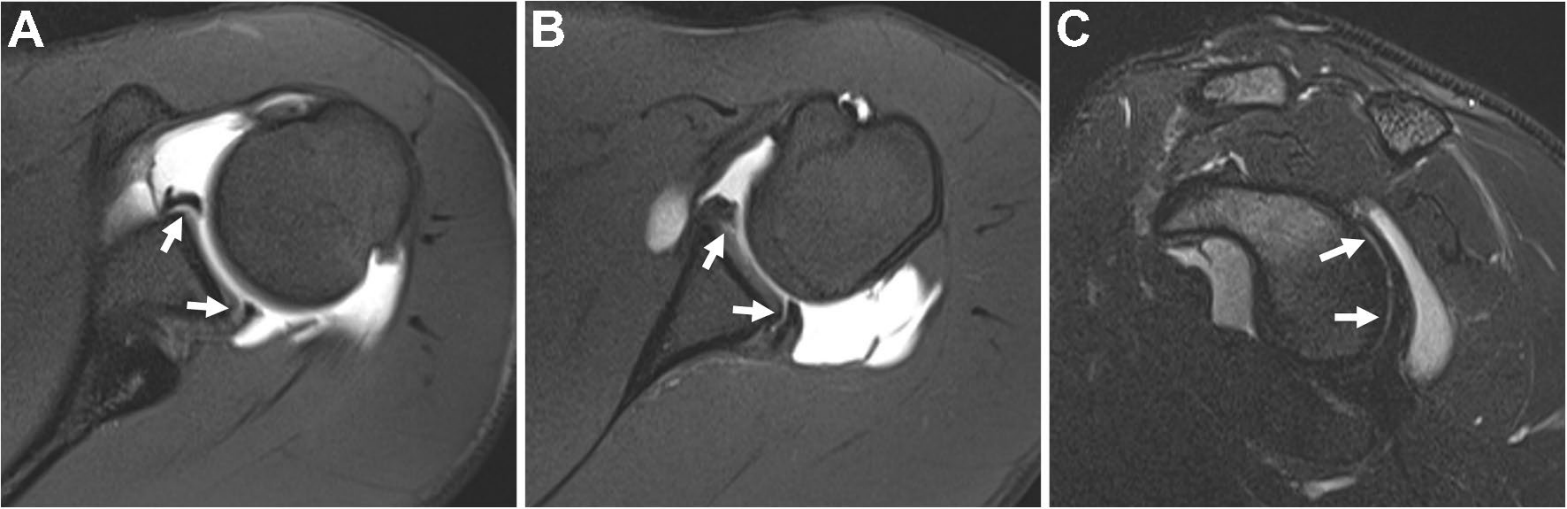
Multidirectional instability. Eighteen-year-old man with shoulder pain. Axial T1-weighted fat-suppressed (A and B) and sagittal T2-weighted fat-suppressed (C) 1.5 T MR arthrogram images demonstrate extensive labral tearing involving both anterior and posterior aspects (arrows)
